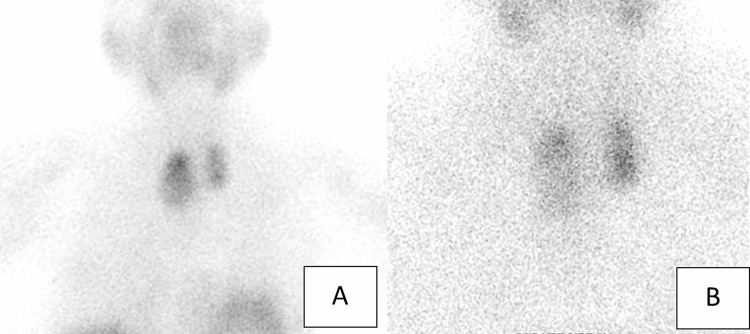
99mTc-sestamibi parathyroid scintigraphy of the neck obtained at the baseline (A) and two hours after (B), showing intense uptake on both sides consistent with hyperactive inferior left and right parathyroid glands.
